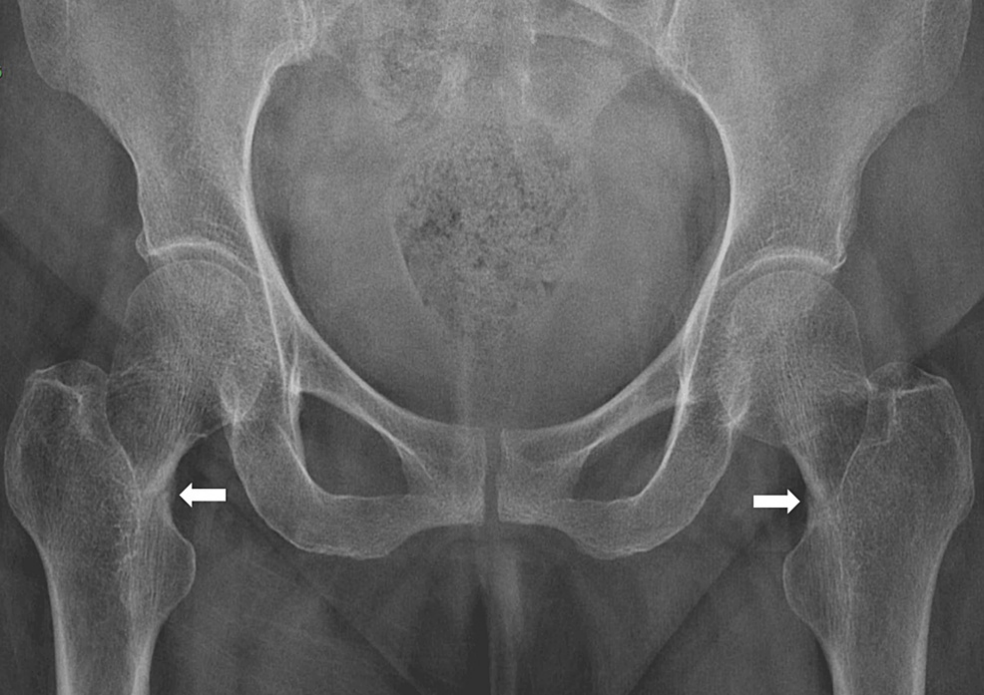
An X-ray of the bilateral hip region showing acute stress fracture (arrows) in the bilateral intertrochanteric region of the femur.
